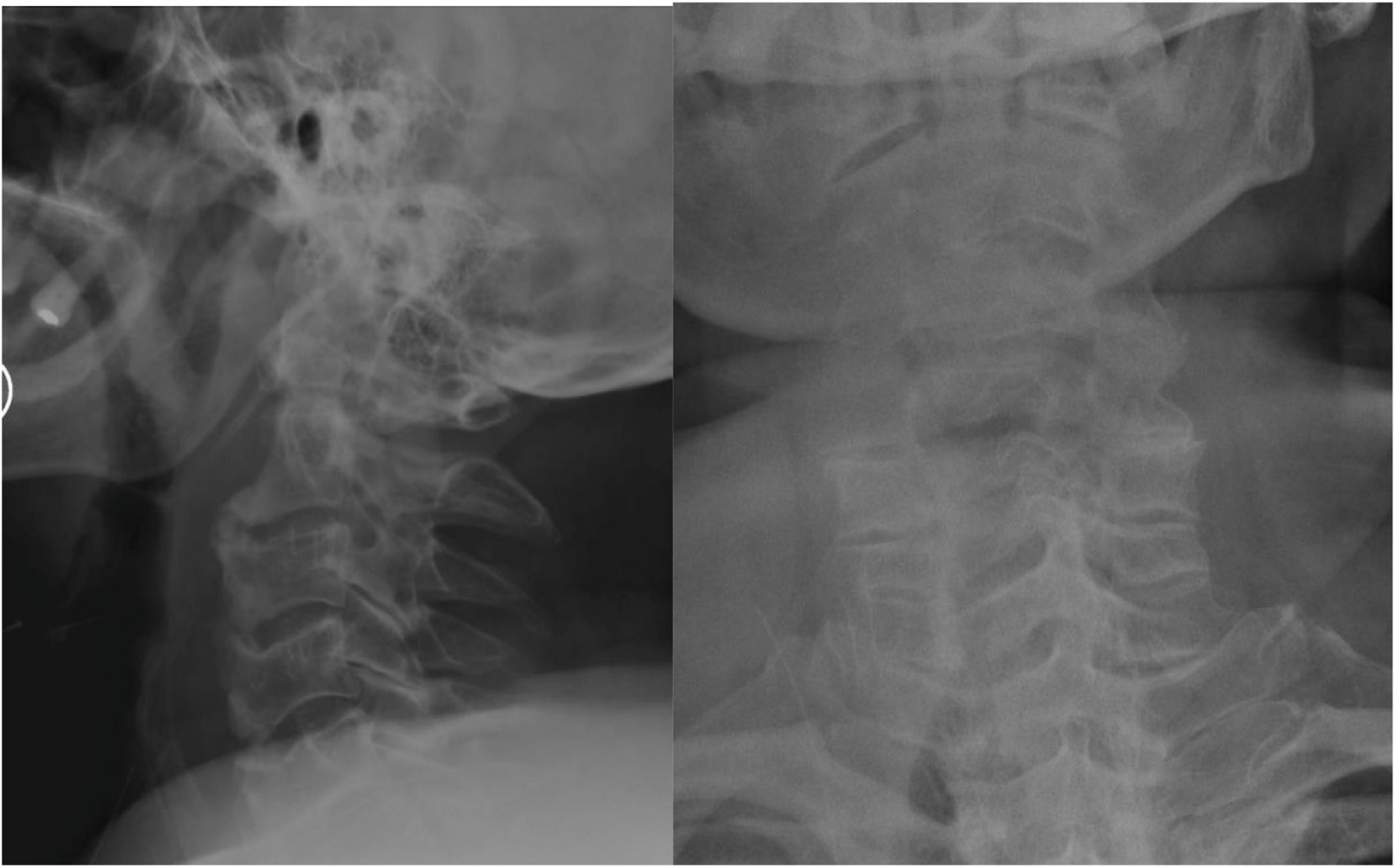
AP and lateral view X-ray of cervical spine after surgery of a 73-year-old man with diffuse idiopathic skeletal hyperostosis in cervical spine and dysphagia.
